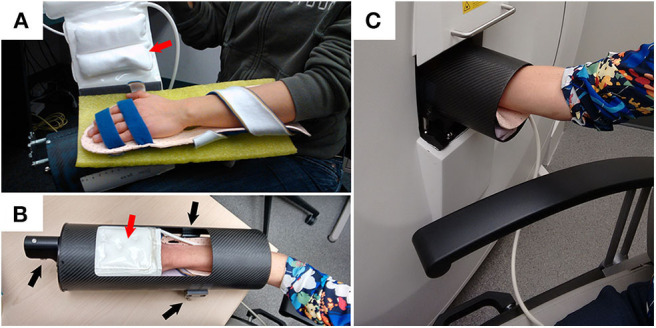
Example demonstrating how a participant is positioned for XtremeCTII scanning. (A) Participant's hand is positioned on a rigid-formed mold, with straps securing the distal and proximal ends of the forearm. An additional x-ray compatible, inflatable, pad-based positioning system (red arrows) is positioned above the hand to further reduce accidental motion during scan acquisitions. (B) The secured appendage is then placed within a cylindrical, annular, carbon-fiber molded sample holder. Using three spherical landmarks (black arrows), and with the participant resting comfortably, the supported participants' hand and sample holder is then securely and accurately placed within the scanner (C).
